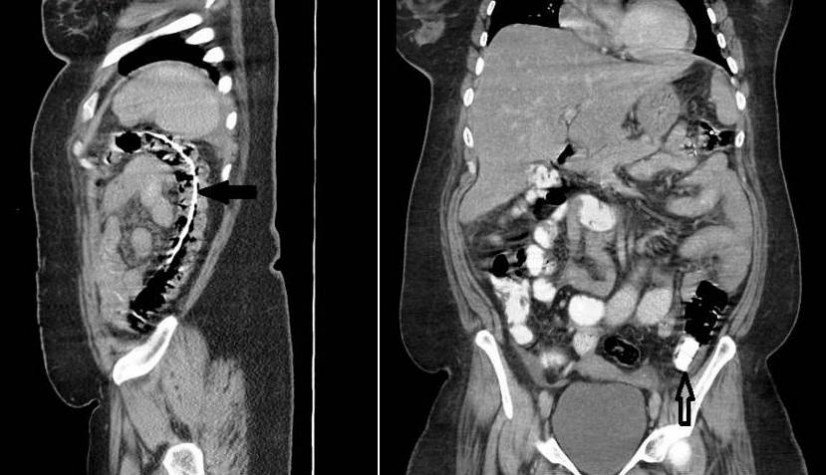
Abdomen sagittal and coronal CT scan showed gastric band connecting tube at splenic flexure and descending colon (thick arrow), the gastric band seen in the distal descending colon (open arrow).
